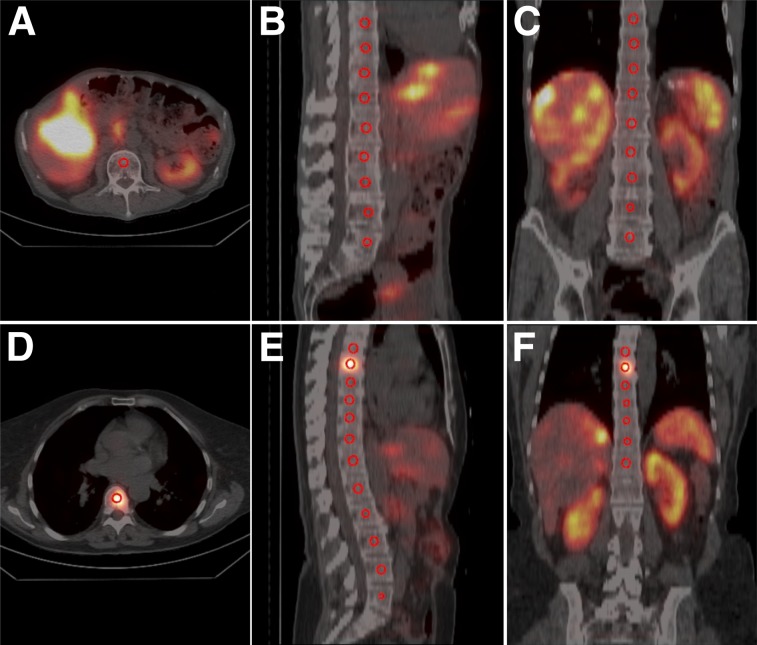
Location of spheric volume of interests in vertebrae. (A–C) Volumes of interest in SPECT/CT images of patient without skeletal metastases. (D–F) Spheric volumes of interest in SPECT/CT images of patient with skeletal metastasis in thoracic vertebra 7.
